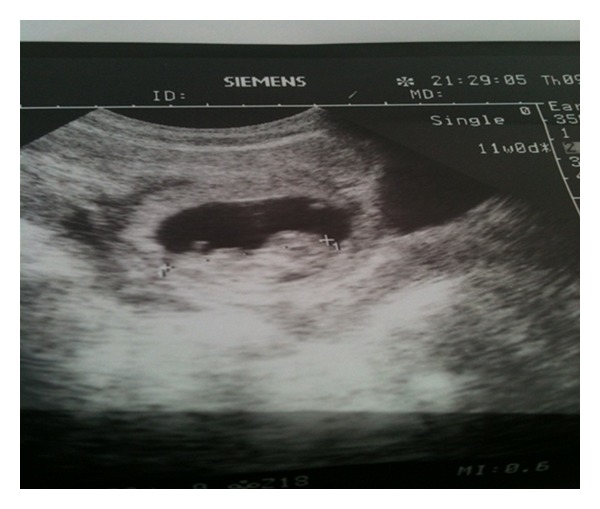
Transabdominal ultrasound showing gestational sac with fetus in the lower uterine segment.
